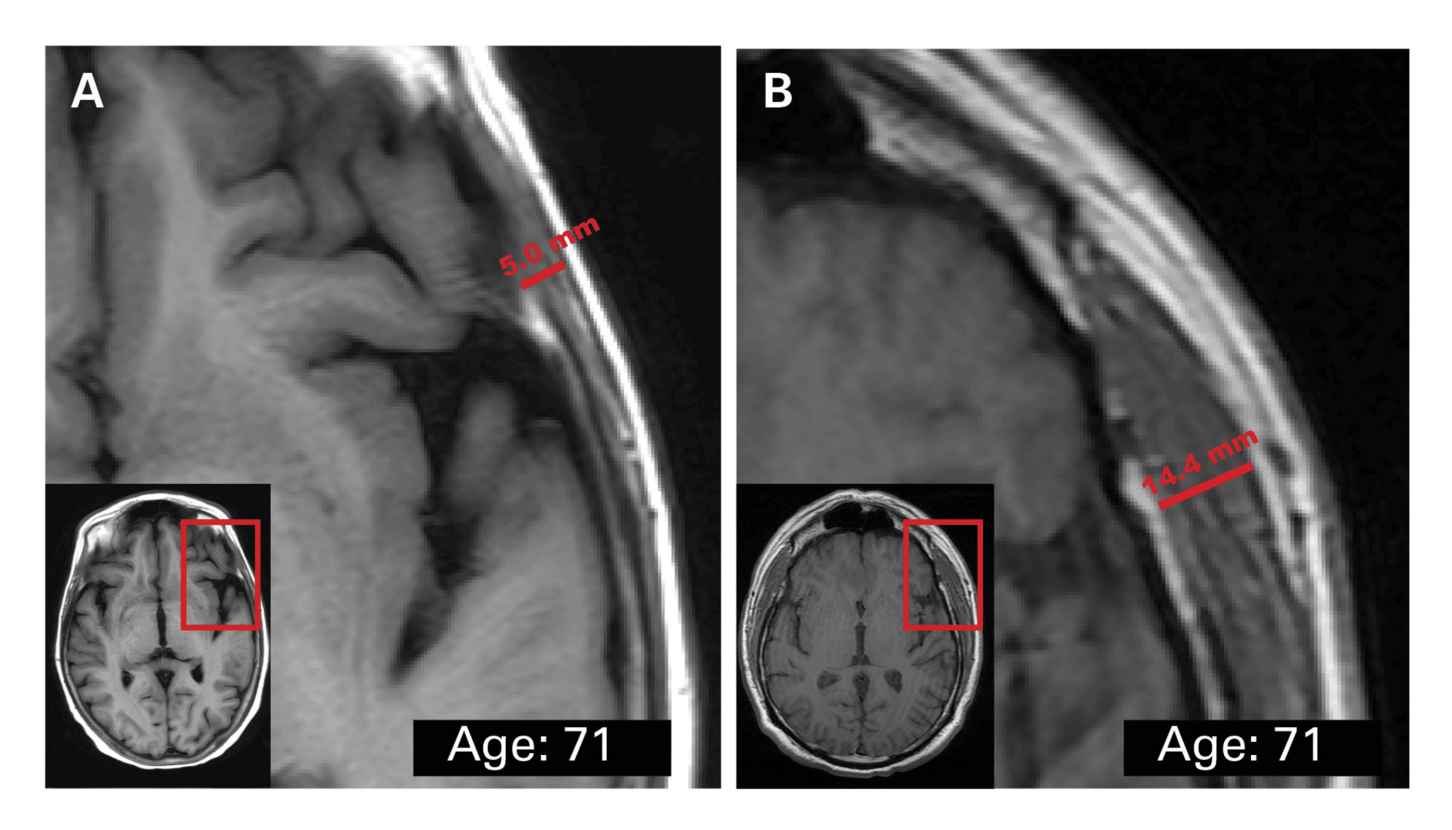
Perioperative measurement of temporalis muscle thickness on T1-weighted axial MRI at initial surgery for the diagnosis of GBM. Figure A depicts a frail 71-year-old male patient with a thin temporalis thickness of 5.0 mm. Figure B depicts a healthier 71-year-old male patient with a thick temporalis thickness of 14.4 mm.
